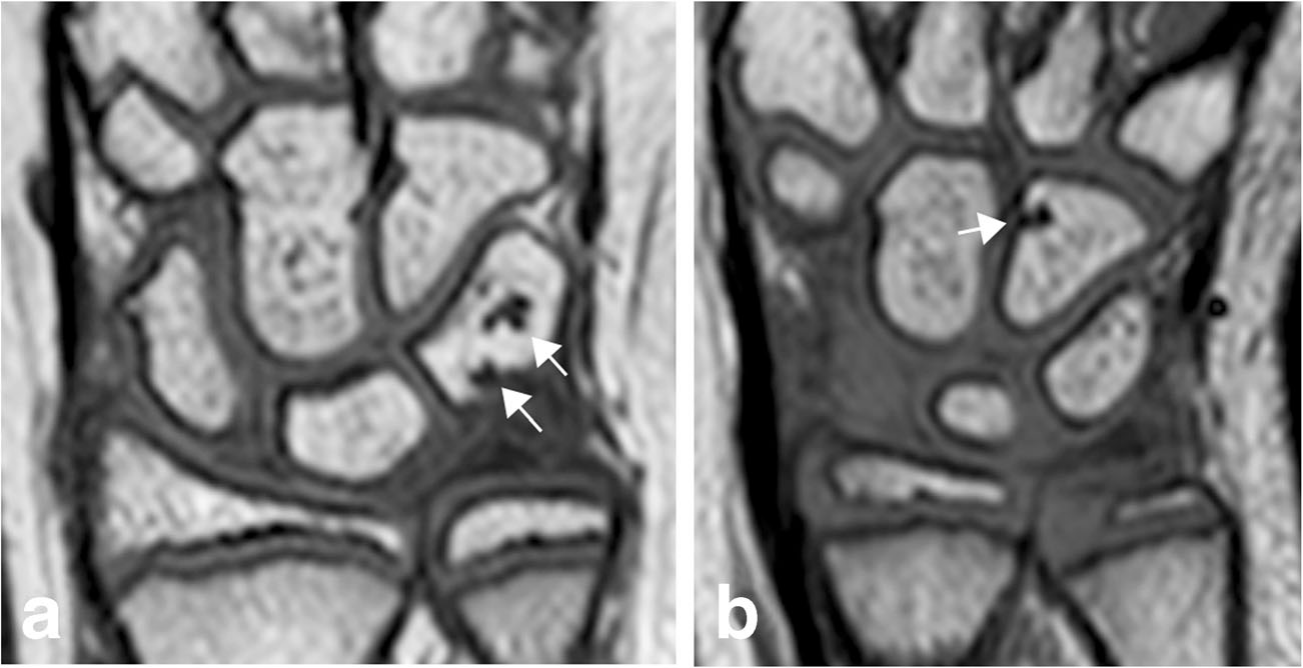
Bony depressions of the carpal bones and proximal metacarpals (arrows) are a normal finding in children and should not automatically be interpreted as erosions. a Coronal T1-weighted image of a 10-year-old healthy girl showing typical normal carpal depressions in the triquetrum. In some areas of the carpal bones bony depressions are more frequently seen in healthy children, e.g. in the radial aspect of the hamate, here shown in an 8-year-old, healthy and asymptomatic girl (b)
